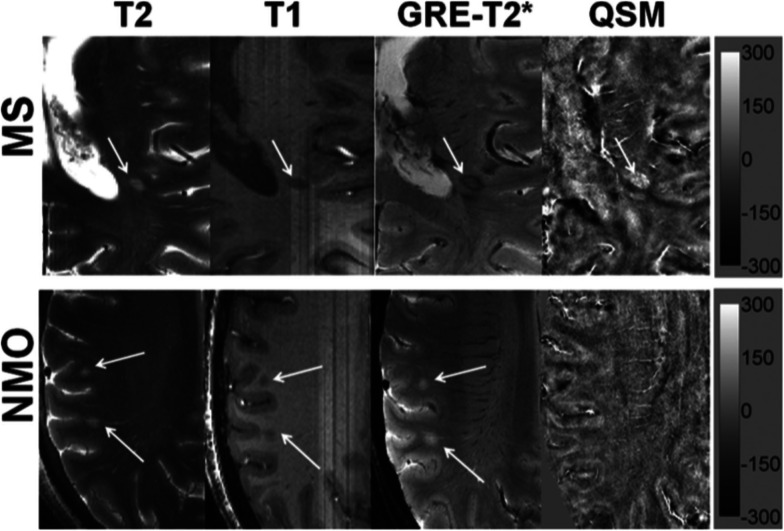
Distinguishing multiple sclerosis (MS) from neuromyelitis optica (NMO) lesions. In the upper row, a patient with multiple sclerosis, from left to right: axial T2-weighted image (T2) with a hyperintense lesion (white arrow) traversed by an ill-defined central venule; the same lesion appears hypointense on a T1-weighted magnetisation-prepared rapid gradient echo image (T1), with hypointense peripheral rim and iso-to-hypointense central core traversed by a well-defined venule on a gradient-echo T2*-weighted image (GRE-T2*), and hyperintense on quantitative susceptibility mapping (QSM). Hypointense signal intensity within the lesion on T2*-weighted image and hyperintensity on QSM suggest iron accumulation. In the lower row, a patient with NMO, from left to right: axial T2-weighted image with two round hyperintense lesions (white arrows); the same lesions appear hypointense on T1, hyperintense on GRE-T2*, and isointense on QSM, thus inconspicuous with iron accumulation. The scale bar is for the QSM image with units of parts per billion. Published with permission from *AJNR Am J Neuroradiol* [74]
